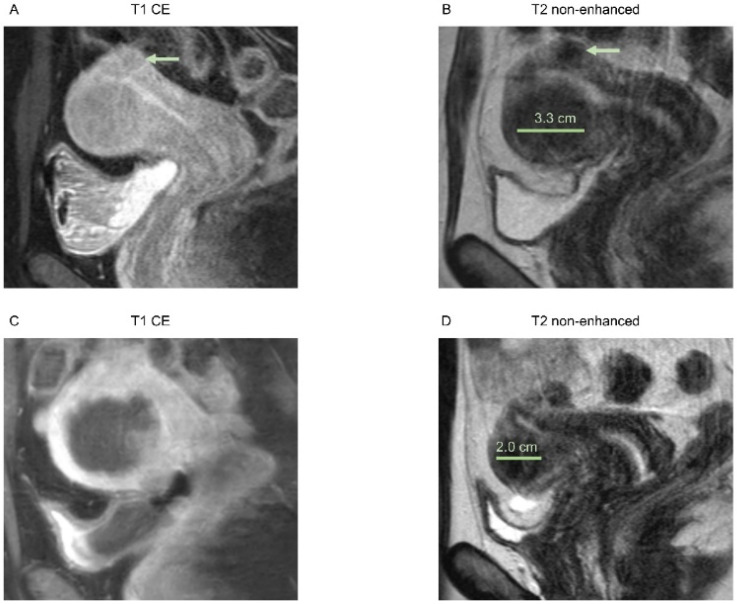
Images of a 39-year-old patient with multiple uterine fibroids in the anterior wall and posterior wall, Funaki grade 2. (A) Contrast-enhanced T1-weighted and (B) T2-weighted images 3 weeks before HIFU treatment of the fibroid in the anterior wall, sagittal reformation. Pre-interventional maximum diameter was 3.3 cm. A smaller subserous fibroid is depicted in the posterior wall (arrow), which could not be reached by HIFU due to bowel loops in the beam path. (C) Immediate post-interventional T1 contrast-enhanced image with a volumetric assessed NPV of 64%, sagittal reformation. (D) T2-weighted image 6 months after intervention with a maximum diameter of 2.0 cm, sagittal reformation.
